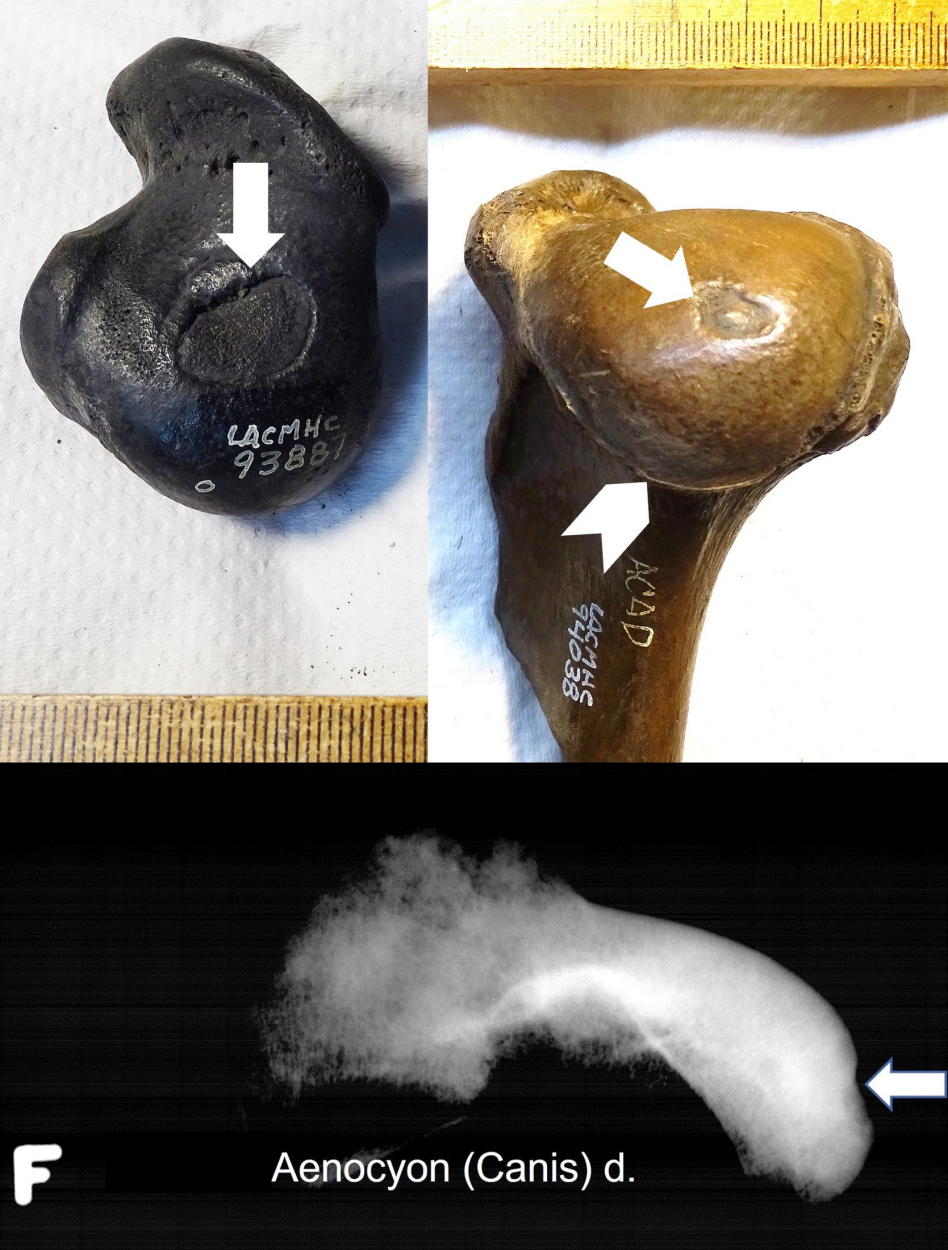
OC/OCD-like joint defects in the proximal humerus of *A*. *dirus* LACMHC 93887 and LACMHC 94038 (arrows) and the radiograph of *A*. *dirus* LACMHC 93743 humeral shoulder joint. The arrowhead indicates a ring of osteophytes indicating osteoarthritis.
